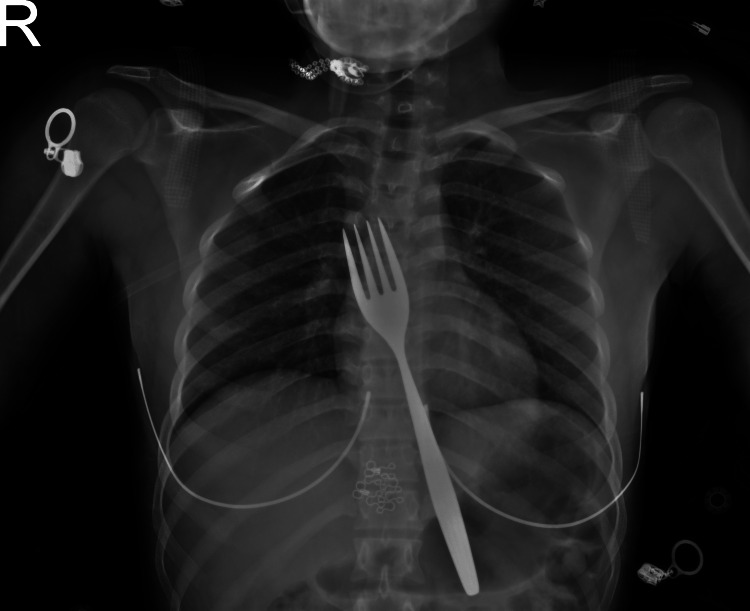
Chest X-ray showing the prongs of the table fork in the esophagus and the handle extending into the gastric lumen.
